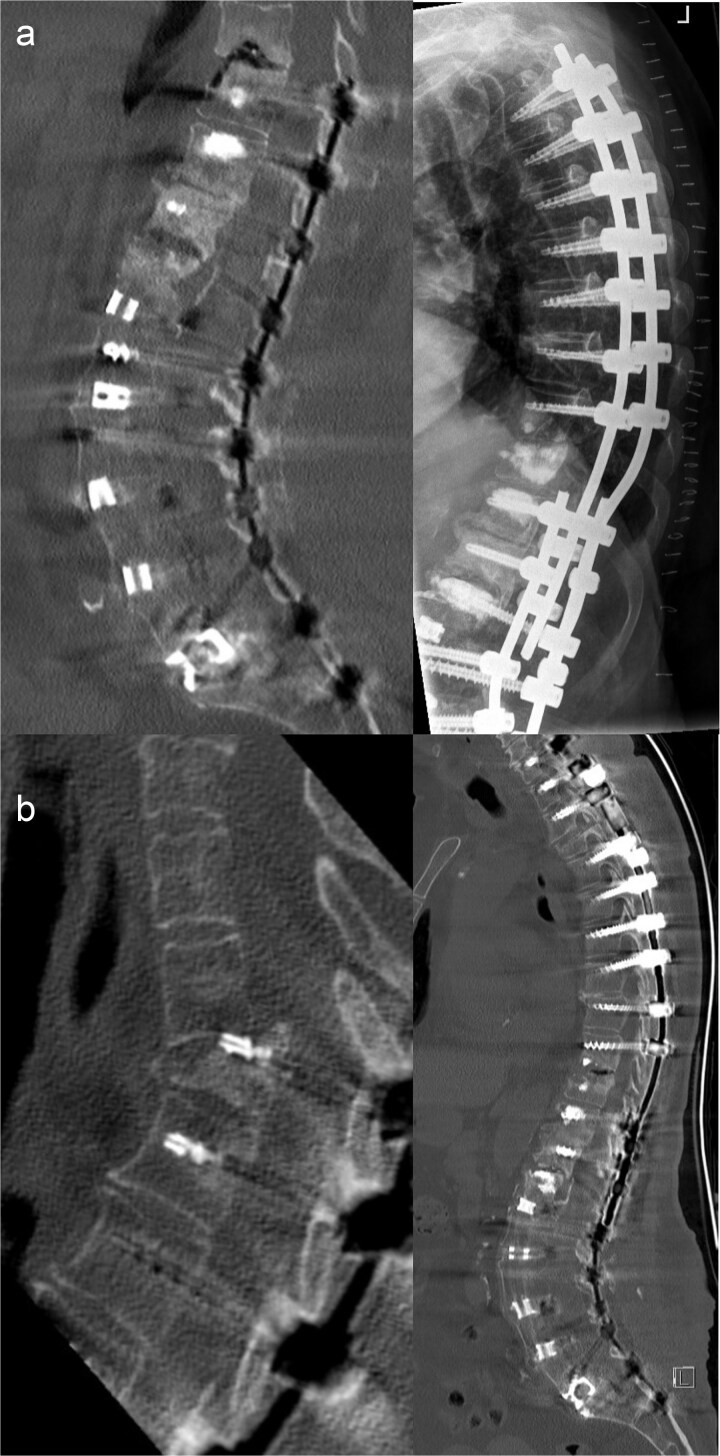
(a) Preoperative magnetic resonance imaging demonstrating an adjacent segment fracture at T9 (left), and postoperative imaging following CT-navigated posterior instrumentation from T3 to T8, including removal of the T10 screw pair and integration of the new construct into the existing instrumentation using cross-connectors (right). (b) Preoperative imaging showing a highly unstable T3 vertebral body fracture (left), and postoperative imaging following urgent CT-navigated posterior fusion from C7 to T2 (right).
